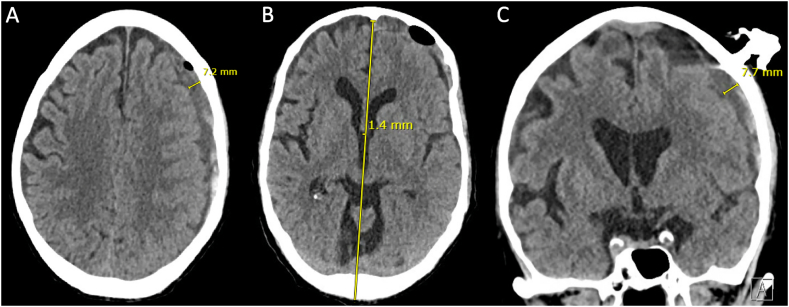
Head CT, after first SEPS. Caption: Head CT after implantation of the first SEPS 17 cm posterior to the nasion and 6 cm left of midline, with evacuation of a superficial chronic component (best seen in Panel C, intracranial and medial to the SEPS drain location). The left convexity SDH improved to 7–8 mm in thickness (Panel A and C), and midline shift improved from 2.3 mm to 1.4 mm (Panel B). CT = computed tomography; SEPS = subdural evacuation port system; SDH = subdural hematoma.
